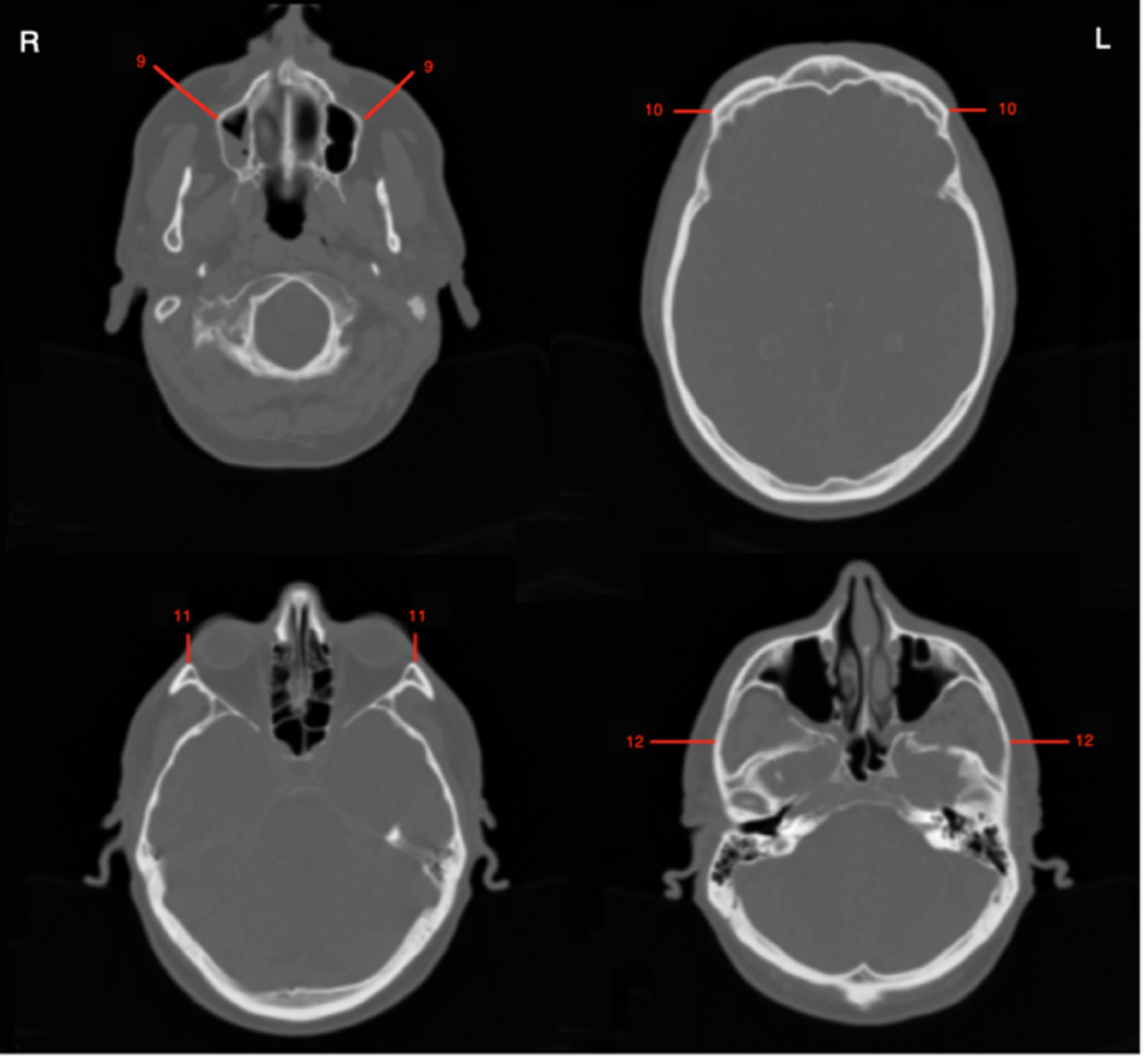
CT slices in the transverse plane, indicating the bilateral cranial landmarks used for the 2D measurement method
